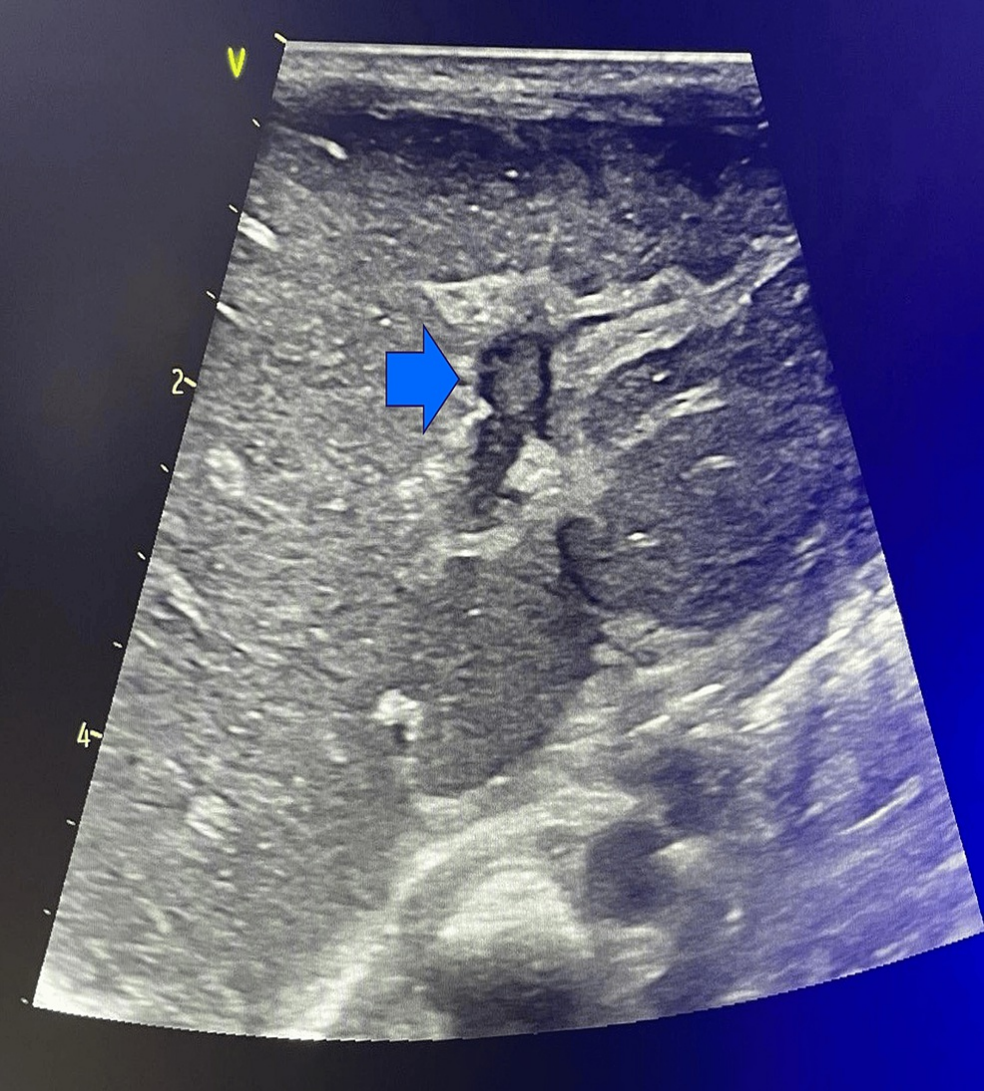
Hepatic ultrasound The arrow points to the thrombus in the left hepatic vein.
